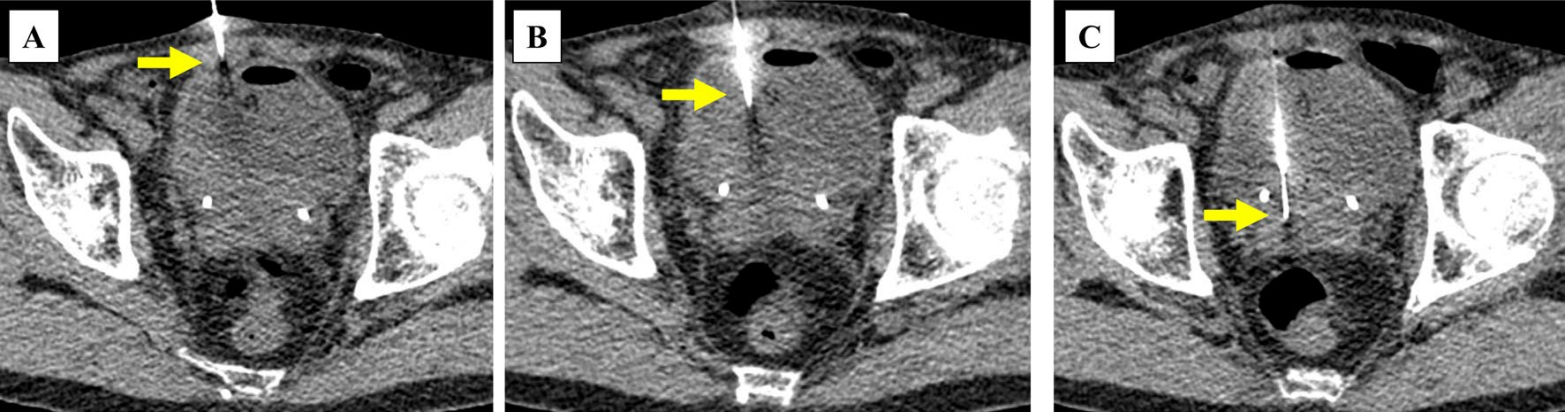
Percutaneous transvesical computed tomography‐guided needle biopsy. After distending the bladder with normal saline, an 18‐G introducer needle was placed into the bladder under image guidance (A, B). Five tissue samples from the bladder mass were obtained using a 20‐G semiautomatic biopsy needle (C). The yellow arrows indicate the position of the needle tip.
